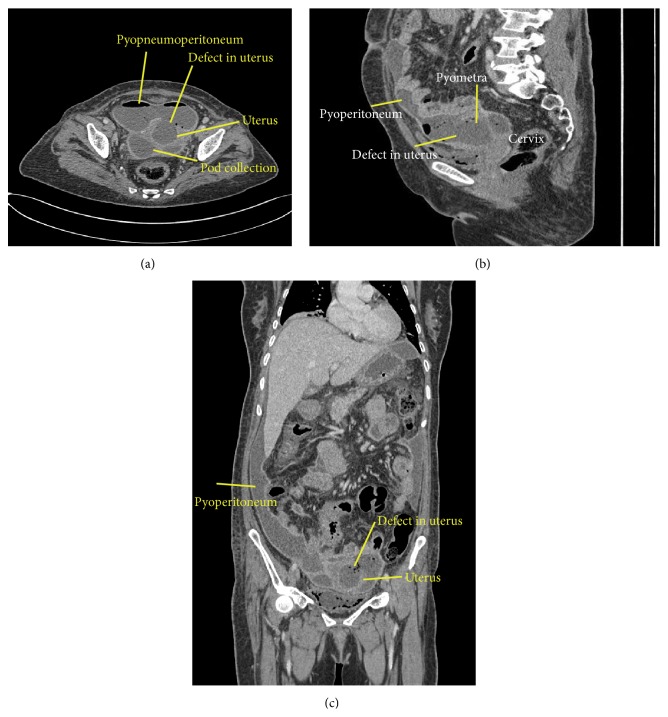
(a) Axial contrast enhanced CT in venous phase reveals pyometra communicating with peritoneal collection through defect in anterior wall of the uterus near fundus. Free nondependant air with smooth peritoneal enhancement suggesting pyopneumoperitoneum. (b) Sagittal contrast enhanced CT in venous phase reveals pyometra communicating with peritoneal collection through defect in anterior wall of the uterus near fundus. (c) Coronal contrast enhanced CT abdomen in venous phase reveals pyometra communicating with peritoneal collection through defect in the uterus near fundus.
